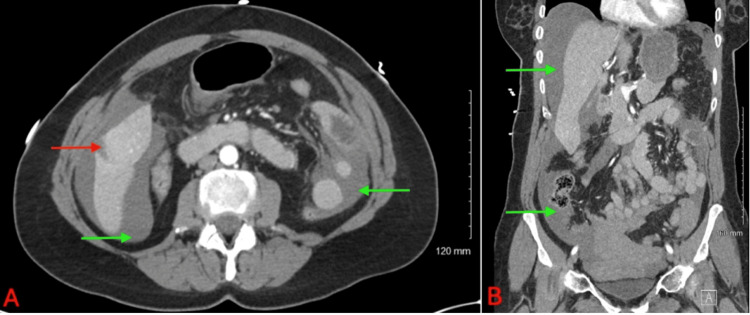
CT abdomen and pelvis (A) Axial CT imaging. Red arrow pointing at a 4 cm nodule in the right lobe of the liver. Green arrows demonstrating diffuse hyperdense peritoneal fluid, 32 HU in the mid to upper abdomen, and 67 HU in the pelvis. (B) Coronal CT imaging. Green arrows demonstrating diffuse hyperdense peritoneal fluid. CT: computed tomography
